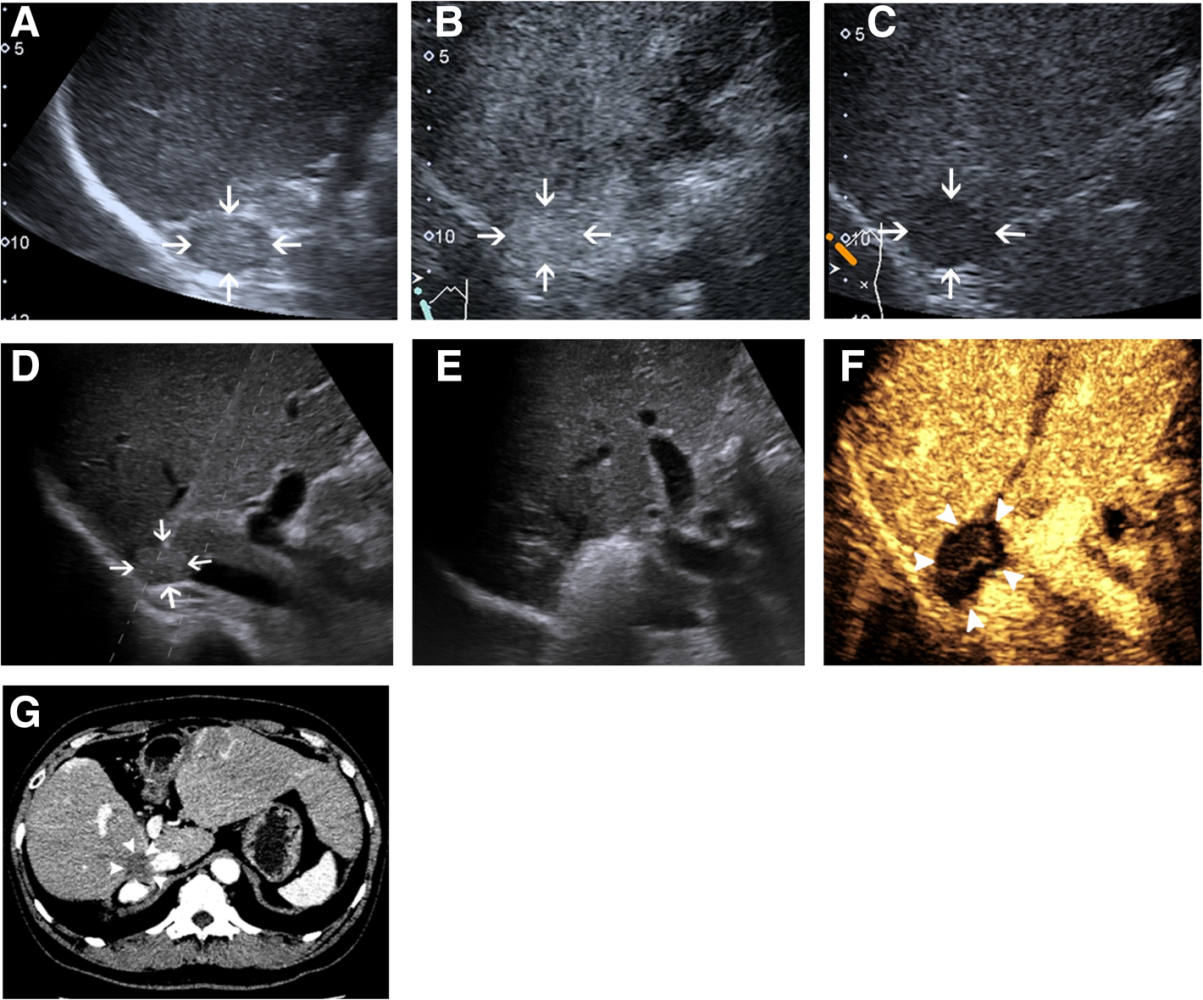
Images in a 46-year-old male patient received radiofrequency ablation (RFA) for adrenal metastasis (AM) originated from hepatocellular carcinoma (HCC). a Pre-interventional B-mode ultrasound showed a round mass at the upper pole of the right kidney (arrow). b and c contrast-enhanced ultrasound (CEUS) before RFA showed obvious enhancement in the early phase (b) and washout in the late phase (c). d Prober placement during the ablation of the right adrenal metastasis. e Tumour appeared completely hyperechoic after the beginning of RFA. f CEUS obtained 1 month after RFA showed no contrast enhancement, suggesting complete ablation. g Contrast-enhanced CT obtained 1 month after RFA showed no contrast enhancement, suggesting complete ablation
